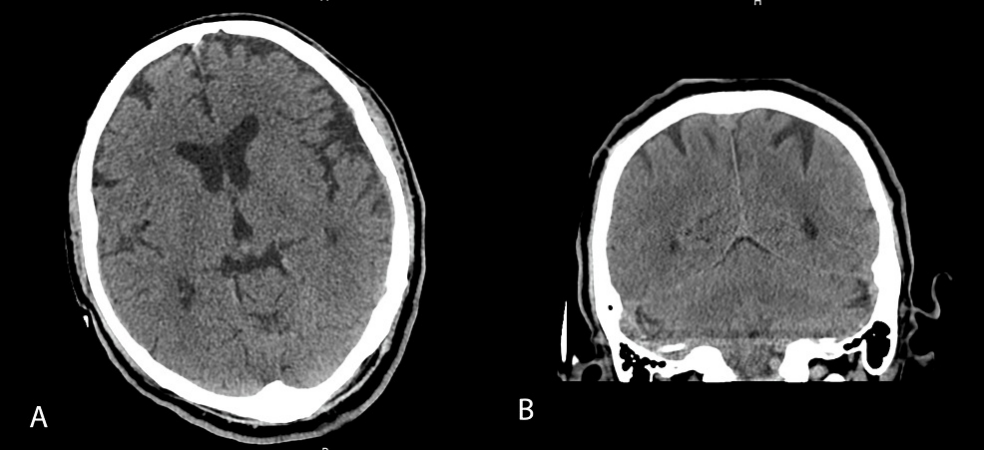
CT scan head without intravenous contrast. A = Transverse view. B = Coronal view
